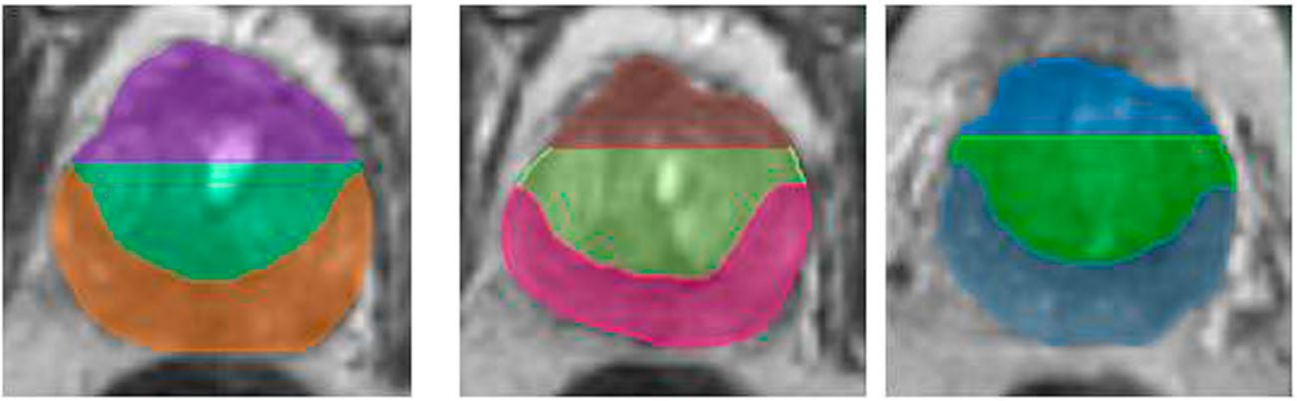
Prostate quadrants. The prostate and the Peripheral Zone (PZ) were manually contoured on the T2-weighted MRI in MIM. Transition Zone (TZ) contour was estimated algebraically by subtracting PZ from the prostate. The prostate is automatically segmented in three sections: apex, mid and base. 3 segments: PZ and two for TZ are automatically generated for each section, resulting in a 9-element 3D grid. Representative T2-weighted axial images in apex, mid and base with the corresponding grid are shown.
